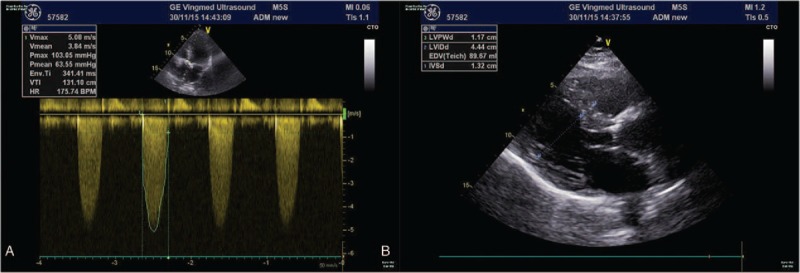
(A) The transthoracic echocardiography before surgery. The echocardiography shows a peak pressure gradient of 103 mmHg. (B) The transthoracic echocardiography before surgery. The echocardiography shows a septum thickness of 1.32 cm.
